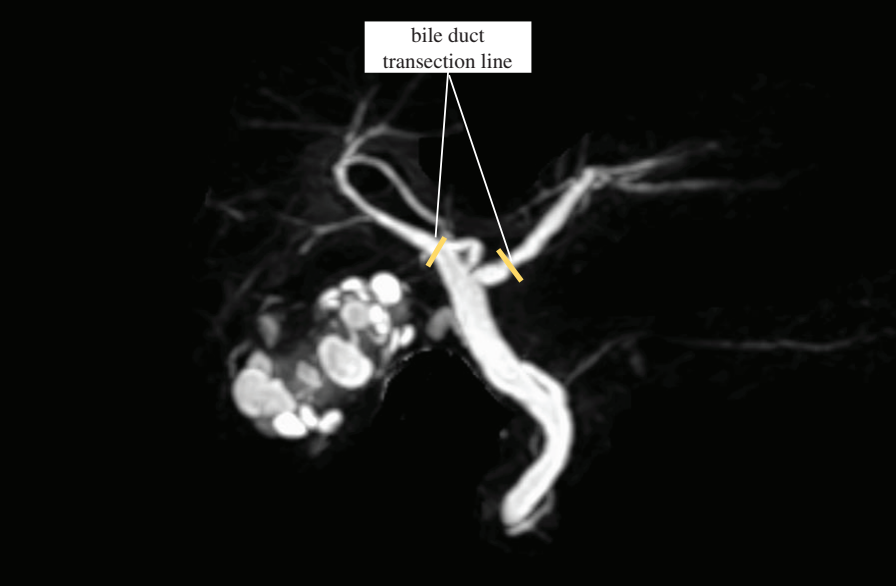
MRI showed that the right hepatic duct ran along the right branch of the portal vein and joined the left hepatic duct.
